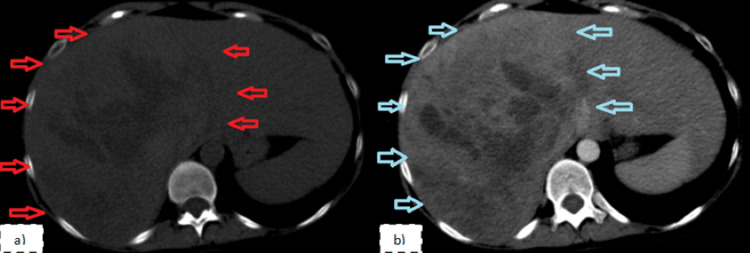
Abdominal CT scan (a) Unenhanced scan. (b) Arterial phase of contrast-enhanced scan. It revealed a giant heterogeneous hepatic tumor with a clear boundary measuring 19 × 14 cm, which completely replaced the right hepatic lobe (red and blue arrows). The intravenous contrast medium showed patchy enhancement. CT = computed tomography
